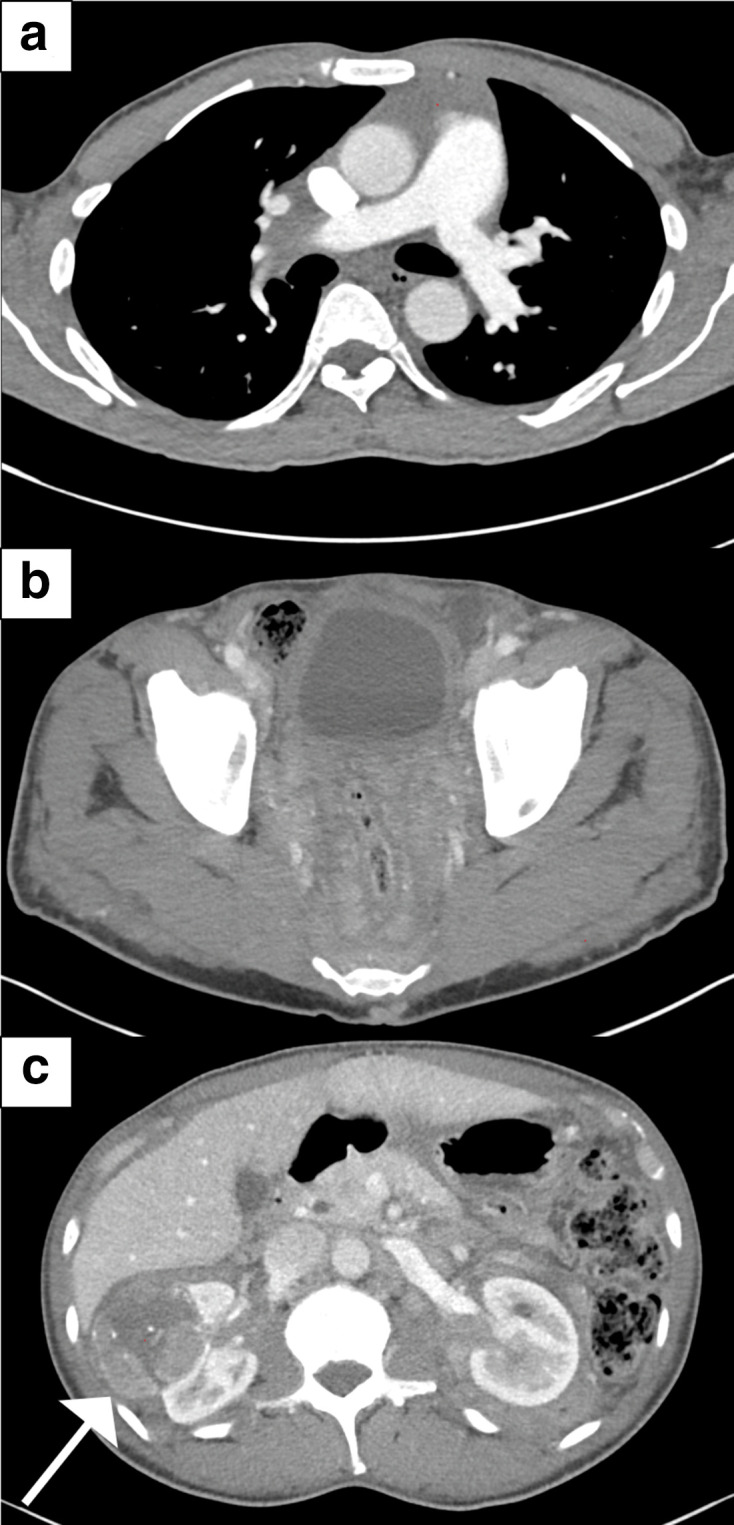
Axial images of the initial CT chest, abdomen and pelvis were remarkable for infiltrative soft-tissue density in the anterior mediastinum (a), retroperitoneum (b), gluteal deep subcutaneous fat (c) and mesorectal fat (c). Additional CT findings of the abdomen demonstrated a complex right renal mass found to be papillary renal cell carcinoma (white arrow).
